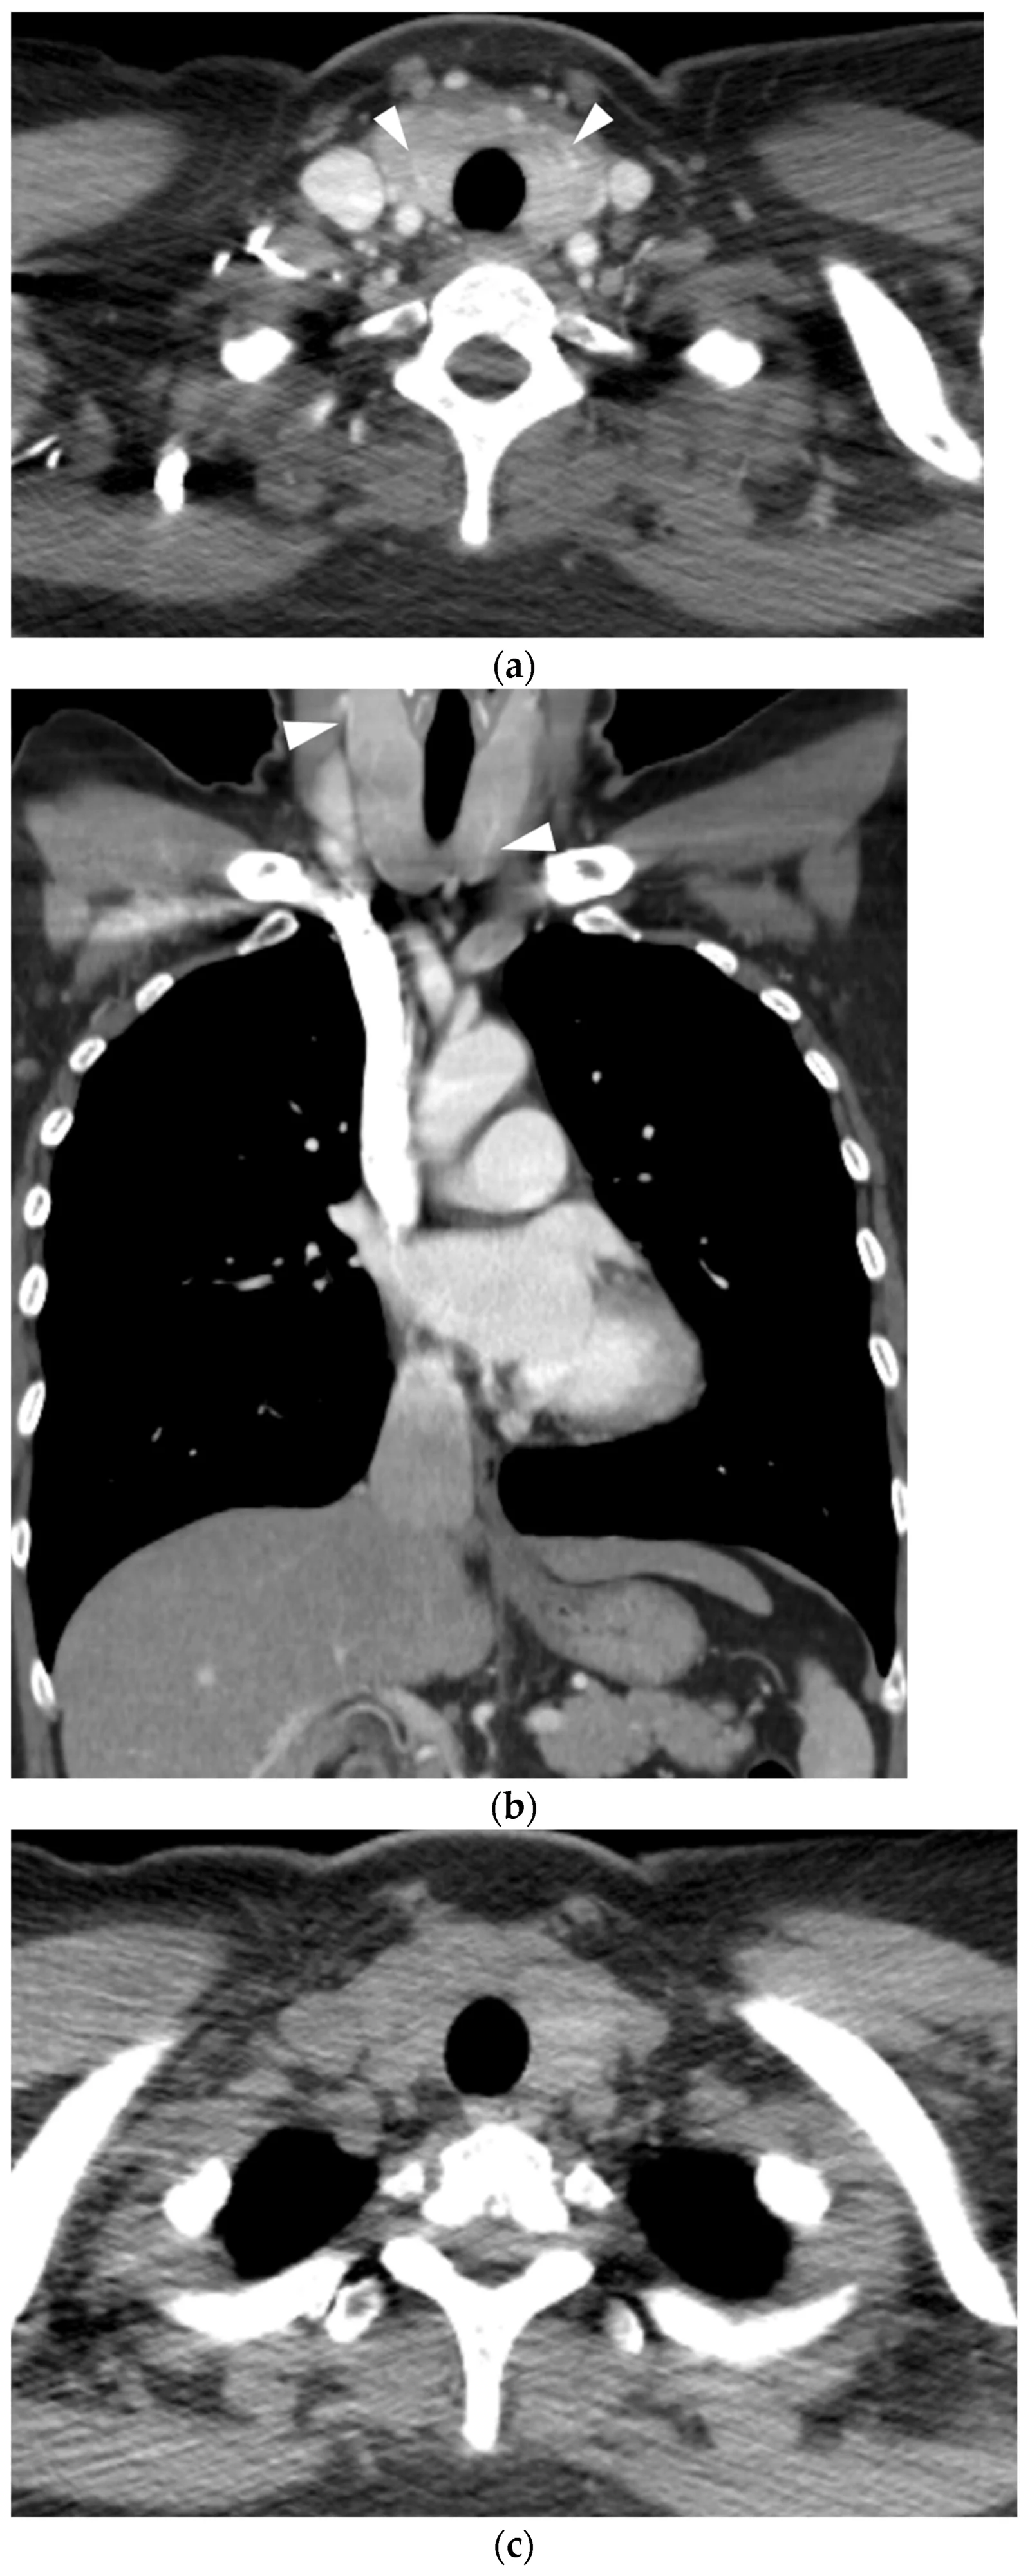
Representative case demonstrating Hashimoto’s thyroiditis on enhanced chest CT in a 50-year-old woman (a,b). Note diffuse thyroid enlargement with heterogeneous enhancement, but enhancing prominent vessels (arrowheads) are noted within the enlarged thyroid glands. This is a characteristic finding in patients with Hashimoto’s thyroiditis as compared to shock thyroid (c). Note diffuse low attenuation of enlarged thyroid glands on the pre-contrast CT image.
